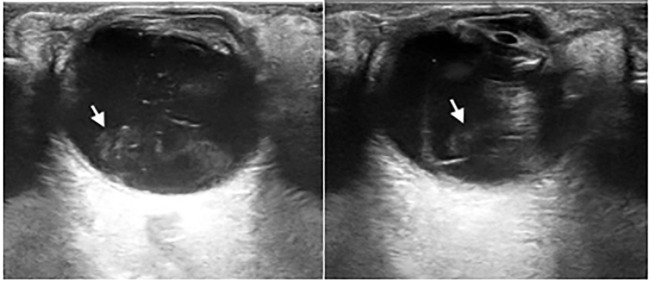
Point-of-care ocular ultrasound demonstrating hyperechoic debris (white arrows) within the vitreous chamber in a patient with endophthalmitis.
